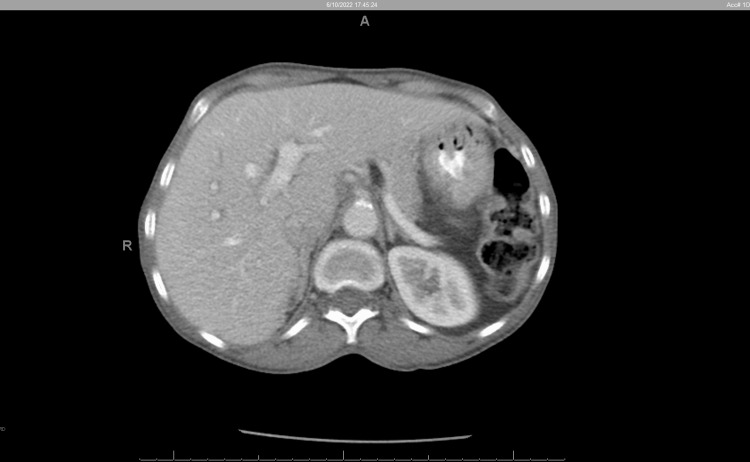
CT abdomen/pelvis obtained pre-operatively. Grossly enlarged liver showing the left lower lobe extending far beyond midline
